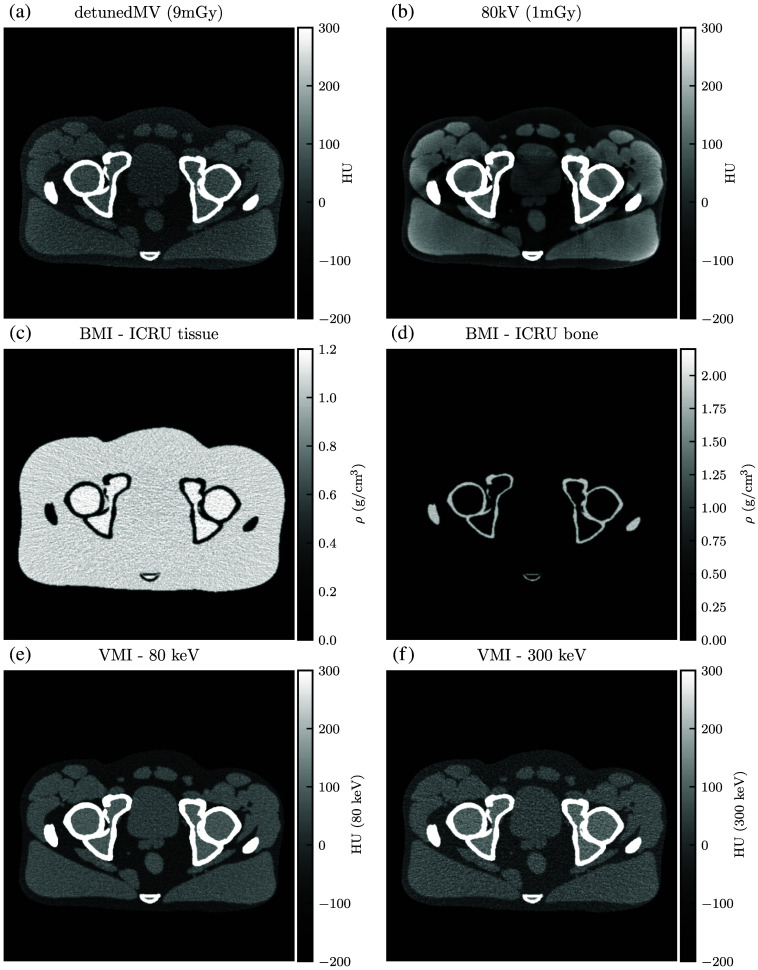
(a) and (b) Single-energy images, (c) and (d) BMIs, and (e) and (f) VMIs for the detunedMV-80 kV DE-CT acquisition of the pelvis with no hip replacement. VMI synthesis corrects for the beam hardening in the 80 kV single-energy image but results in increased noise from the detunedMV single-energy image.
